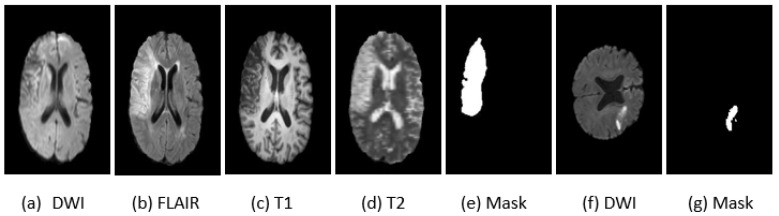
Examples of stroke images and corresponding masks from the SISS 2015 (a–e) and ISLES 2022 (f,g) datasets Across various MRI modalities.
